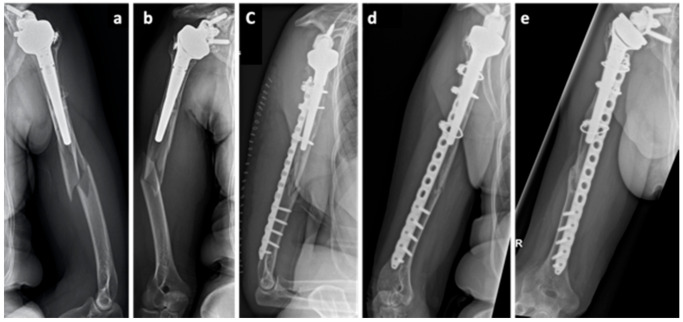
Case 3, x-ray showing the initial periprosthetic humeral fracture (UCS I.1B1) (a,b), the initial postoperative radiograph (c), delayed union 6 months after trauma (d) and bone union at the end of the teriparatide treatment, 12 months after trauma (e).
